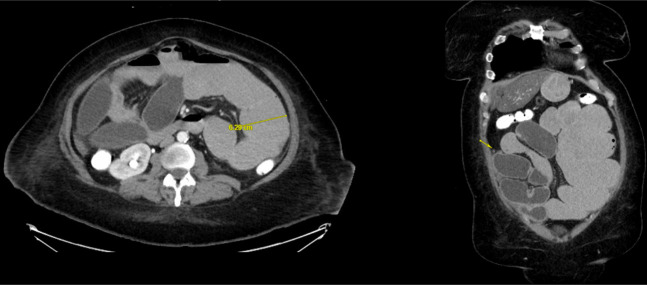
Computed tomography of the abdomen and pelvis showing multiple dilated small bowel loops measuring up to 6.3 cm and a transition point in the right lower quadrant, indicating a possible closed-loop obstruction in the ileum.
